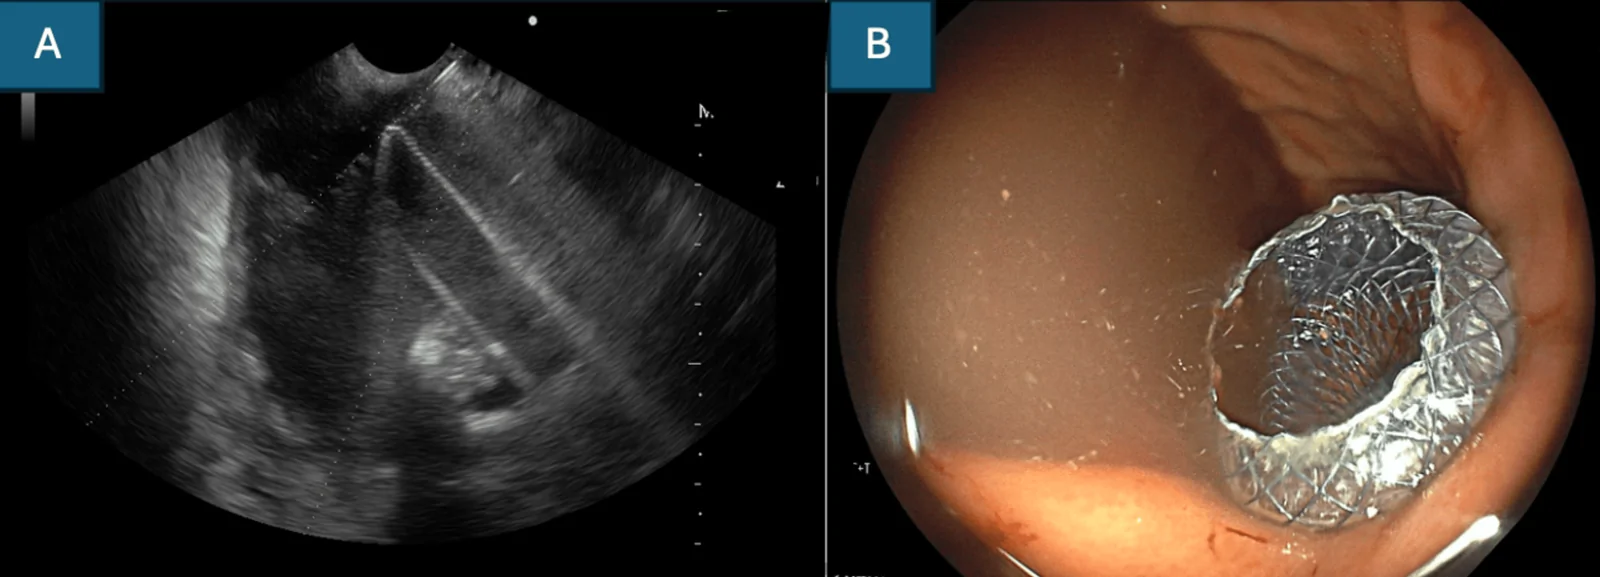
Walled-off necrosis (WON). (A) Endoscopic ultrasound (EUS) view with the stent in situ. (B) Necrotic content drained into the gastric cavity.
